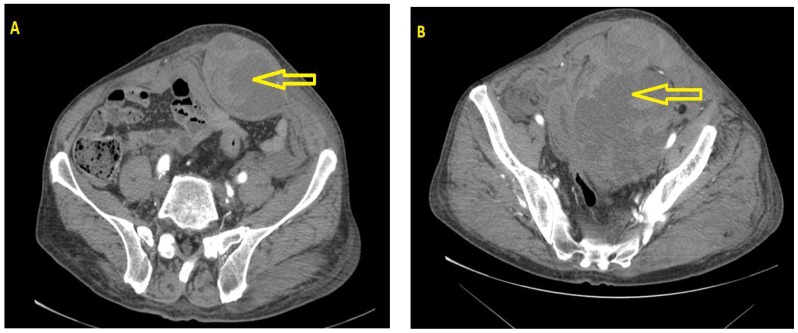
Type III rectus sheath haematoma (RSH) according to classification of Berna et al. [4]: (A) haematoma of rectal abdominal muscle; (B) haematoma proliferates to prevesical and retroperitoneal space. (The computer tomography (CT) scan images are from Department of Radiology, Lithuanian University of Health Sciences, Kaunas Clinics).
